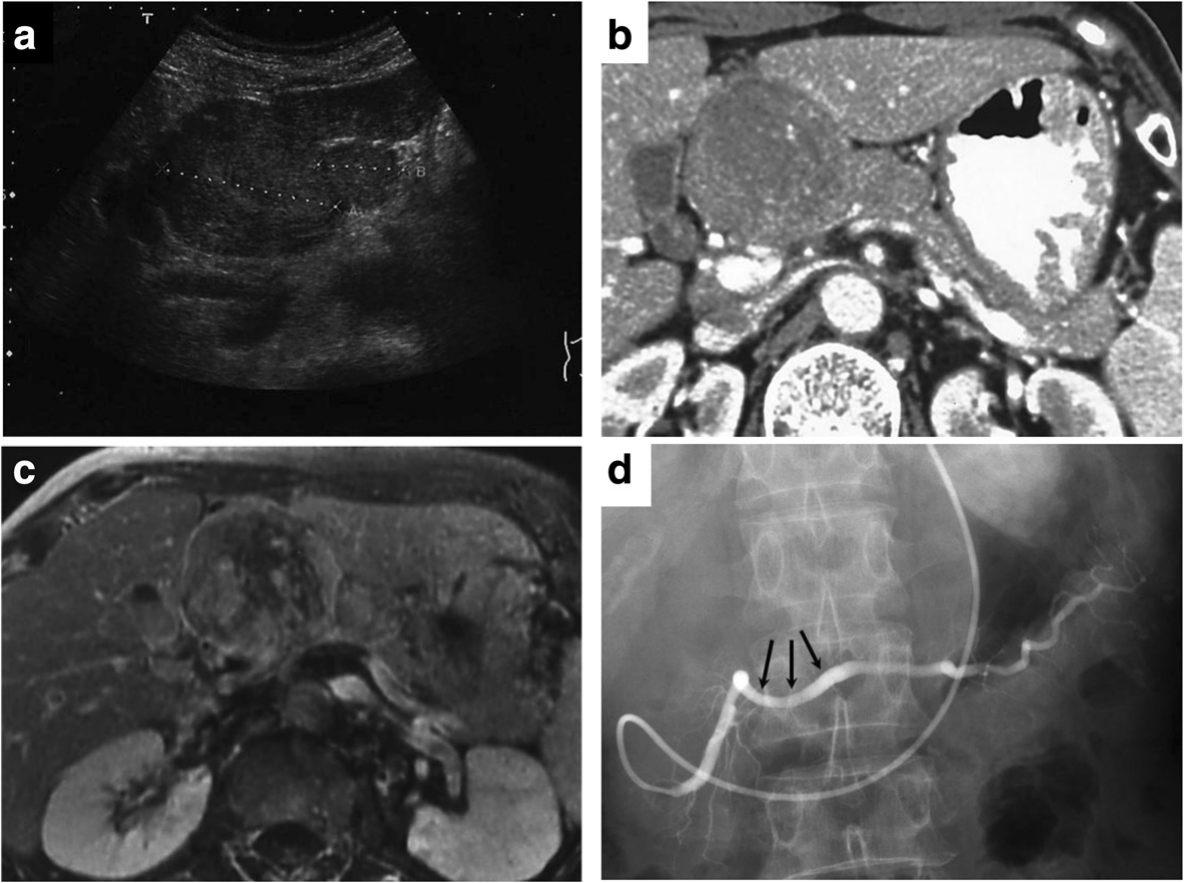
Imaging studies. a Ultrasonography showed that gourd-shaped hypo- and iso-echoic mass, 56 mm in size, was detected in the pancreatic head and body. b Enhanced computer tomography showed that solid well-circumscribed low-density mass, 7 cm in diameter, was located in the pancreatic head and body. c Heterogeneously enhanced mass was detected in the pancreatic body by gadolinium-enhanced MRI. d Endoscopic retrograde pancreatography (ERP) showed that main pancreatic duct was slightly deviated downward because of locoregional pressure effect, but direct invasion to the main pancreatic duct was not evident
